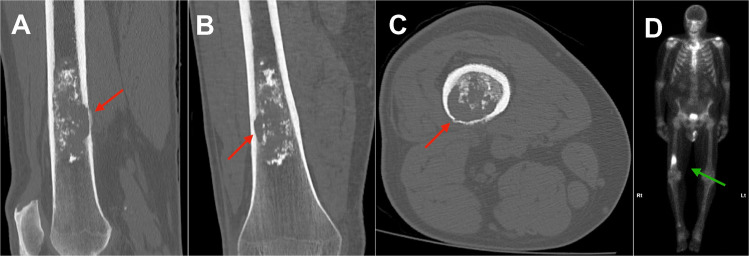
(A–D) CT demonstrates the biphasic pattern of the mass with the characteristic ‘ring and arc’ pattern of cartilage matrix mineralisation of the intramedullary lesion, and focal lytic areas with deep endosteal scalloping posteriorly (red→); bone scan [D] demonstrating uniformly avid tracer uptake in the solitary bone lesion in the distal femoral shaft (green→) in comparison to the anterior iliac crest
